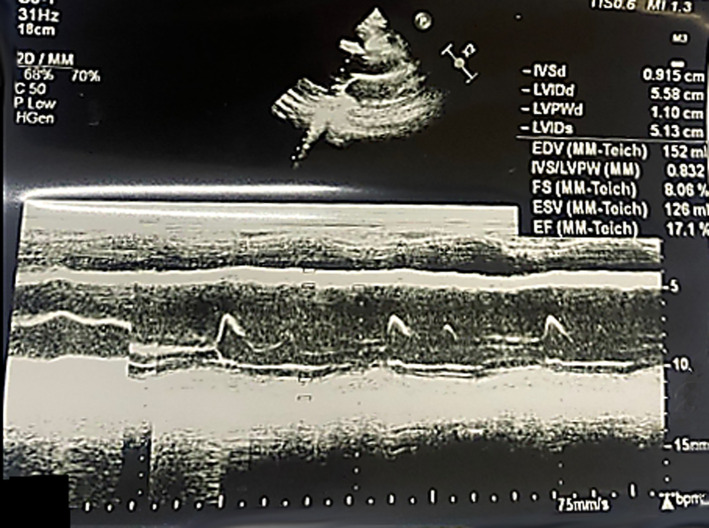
transthoracic echocardiography in long-axis view showing left ventricular ejection fraction of 17.1%
